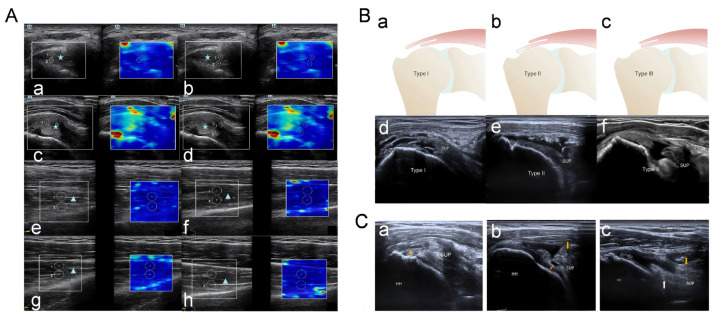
(A): Ultrasound image of the supraspinatus tendon and muscle measured by SWE. (a,b): Natural position SWE measured SWV at four sites of the supraspinatus tendon; (c,d): modified crass position SWE measured SWV at four sites of the supraspinatus tendon; (e,f): Natural position SWE measured SWV at four sites of the supraspinatus muscle; (g,h): modified crass position SWE measured SWV at four sites of the supraspinatus muscle. Pentagram: residual tendon after tear of supraspinatus tendon. Triangle: supraspinatus muscle [127]. (B): Graphical representations and ultrasound images of three shapes of delaminated tears. (a,d): Type I tears, showing greater retraction of articular layer; (b,e): type II tears, showing greater retraction of bursal layer; (c,f): type III tears, showing equal retraction of both layers. (C): Ultrasonographic signs of delaminated tears of supraspinatus tendon in longitudinal plane. (a): Anechoic horizontal linear splitting of tendon (yellow triangle); (b): Anechoic horizontal linear splitting of tendon (yellow triangle) with the retraction of bursal layer (yellow arrow); (c): Bursal layer (yellow arrow) more retract than articular layers (white arrow). HH, humerus head; SUP, supraspinatus [130].
